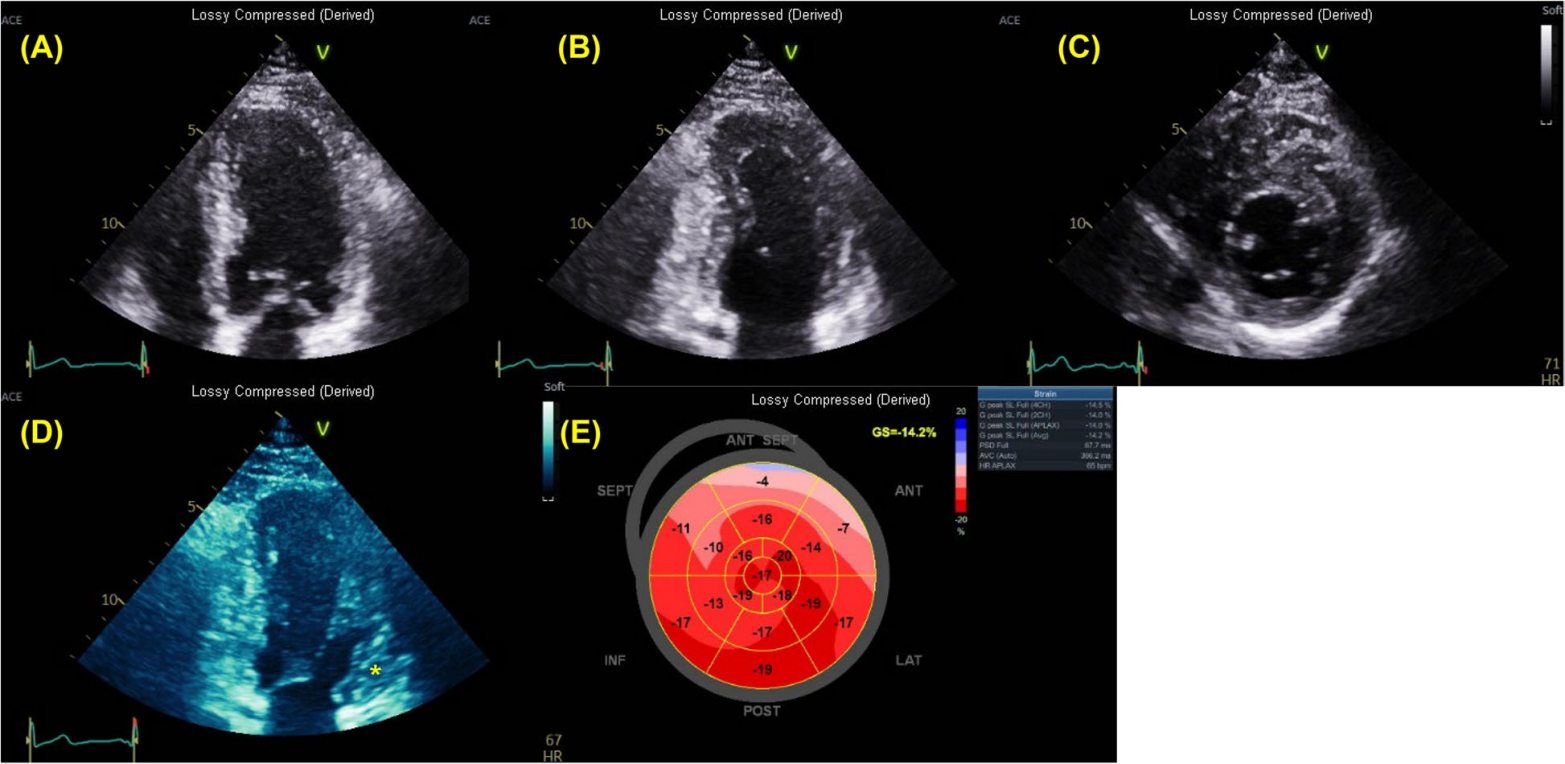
Transthoracic echocardiography of 38-year-old male patient with hypertrophic cardiomyopathy. No definite myocardial hypertrophy is observed in the (A) apical four-chamber and B two-chamber views. C However, thickening of the basal anterior wall is noted in the parasternal short-axis view. D A slightly adjusted apical two-chamber view demonstrates basal anterior wall hypertrophy (asterisk). E Longitudinal strain is reduced in the basal anteroseptal and anterior walls
